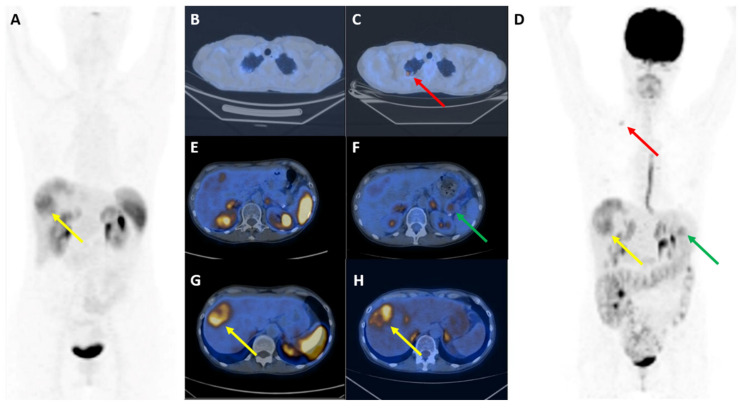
[^68^Ga]Ga-DOTANOC (A,B,E,G) and [^18^F]FDG (C,D,F,H) images in a G2 panNET patient studied for staging. Discordant pattern of uptake can be documented at lung level (transaxial fused images and MIP (B–D): red arrows shows FDG-only positivity), at pancreatic primary level (transaxial fused images (E,F): green arrows show FDG-only positivity), and liver level (A,D,G,H: yellow arrows show different distribution of both tracers within the same lesion).
